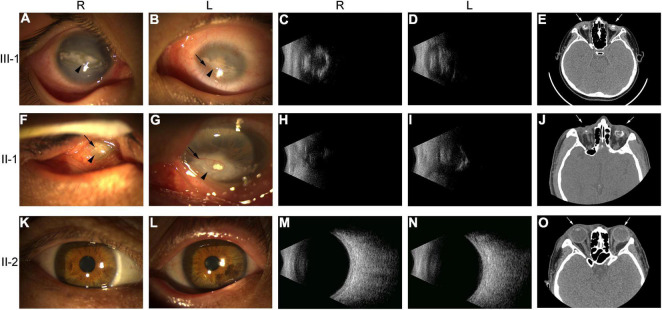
Ophthalmologic examination of members III-1, II-1, and II-2 of the ND family. (A,B,F,G,K,L) Anterior segment photography of III-1, II-1, and II-2. Black arrows indicated neovascularization; arrowheads indicated keratoleukoma. (C,D,H,I,M,N) Vitreous supersonic inspection. (E,J,O) CT inspections of the ears and eyes. White arrows indicated eyes. R, right eye; L, left eye.
